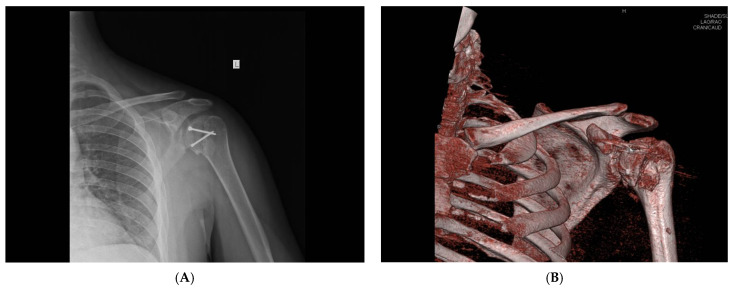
Postoperative X-ray (A) and three-dimensional CT view (B) of the operated shoulder (photo from the personal archive of intraoperative photos of the author).
